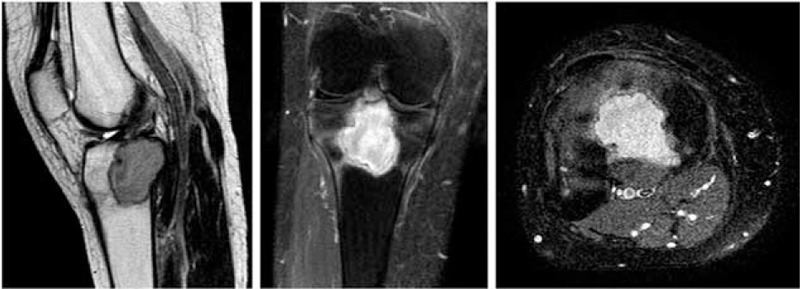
Magnetic resonance images showed the sharply defined lumps and abnormal signal intensity. The lesion is located eccentrically in the posterior aspect of the right proximal tibia and extends close to the articular surface.
